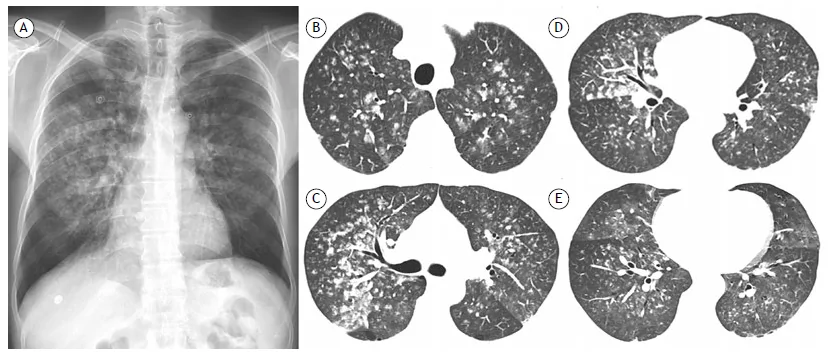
Chest radiograph (A) showing non-homogeneous opacities, predominantly in the middle thirds of the lungs. Axial chest CT images (B-D) showing ground-glass nodules and areas of consolidation and ground-glass attenuation, distributed diffusely throughout the lungs.
